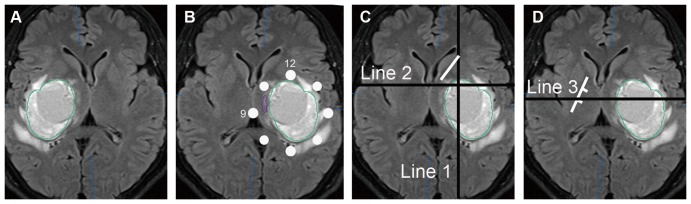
Demonstrating the method of determining the pyramidal tract position and how to draw three lines for classifying the tumors. A. An axial T2 FLAIR image crossing the foramen of Monro. A tumor is shown at the right basal ganglion. B. The tumor was mirrored to the left. The white points around the tumor indicate the o'clock scale with the 12 o'clock ahead and nine o'clock on the medial side. The PT, depicted by the purple line, was located between 12 and nine o'clock. C. The short, white line indicates the anterior limb of the internal capsule. The vertical, black line indicates line 1, which crossed the white line's vertex point. The horizontal black line was line 2, which crossed the foramen of Monro. D. The short, white line indicates the posterior limb of the internal capsule, which was divided equally into three parts. The horizontal black line indicates line 3, which crossed the marker between the middle and lateral thirds of the white line.
